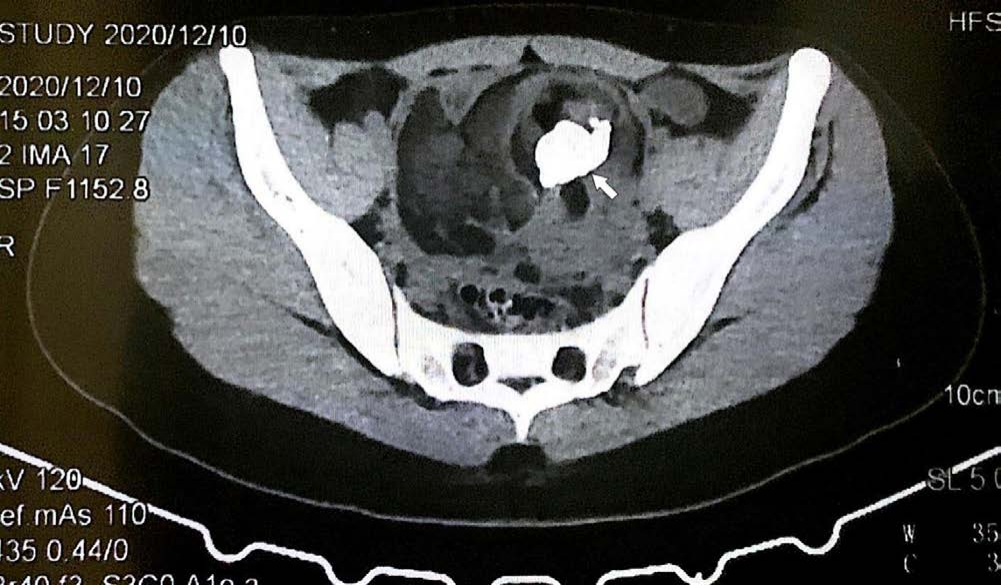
Preoperative abdominal CT images of the patient, the white area indicated by the white arrow is the lesion location, where fat density and high-density shadows were seen.
